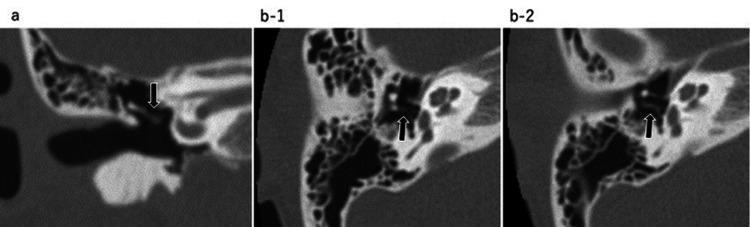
Temporal bone CT at age seven. a: Coronal view: The image of the long process of the incus is obscure (black arrow). b: Axial view: The image of the long process of the incus is obscure (black arrows). There is no sign of soft tissue densities indicating congenital cholesteatoma. CT: computed tomography
